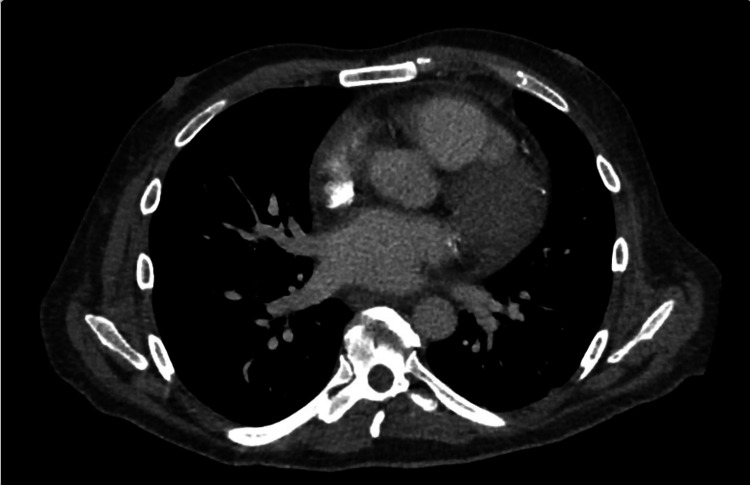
Initial CT Angiography (subsequent slice) Mild pericardial effusion surrounding the heart as seen in the increased thickness of the pericardial border with fluid-density attenuation.
